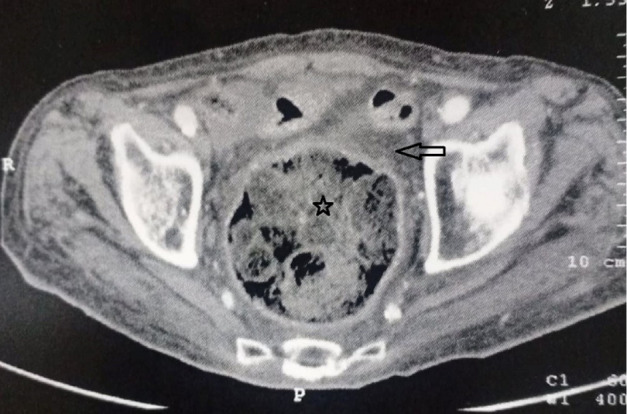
abdominal CT revealing peri-rectal fat standing (arrow) dilation of the rectum, site of several faecalomas (star)
